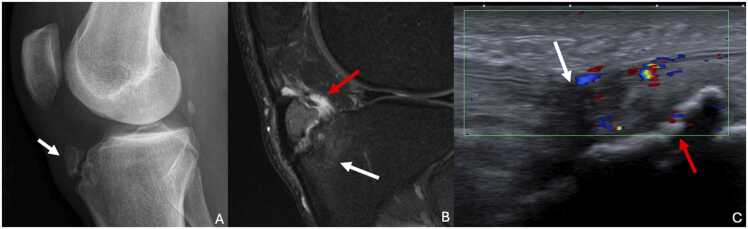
A,B. Osgood-Schlatter disease of the left tibia in a 37-year-old man presenting with pain and swelling of the anterior aspect of the left knee, with no notion of trauma. C. Osgood-Schaltter disease of the left tibia in a 11-year-old boy (different patient). A. Profile radiography showing avulsion of secondary ossification nucleus of the anterior tibial tuberosity (ATT) (white arrow). Corticalisation of margins is in favour of an old avulsion. B. T2-weighted fat-suppressed MR image in the sagittal plane showing bone edema of this secondary ossification nucleus and ATT (white arrow) with inflammatory signal of adjacent soft-tissue, more specifically infrapatellar fat pad (red arrow). C. Doppler ultrasound image in the sagittal plane showing irregularities of ATT (red arrow) with hypoechogenicity (white arrow) and hyperhemia of the distal enthesis of patellar tendon.
